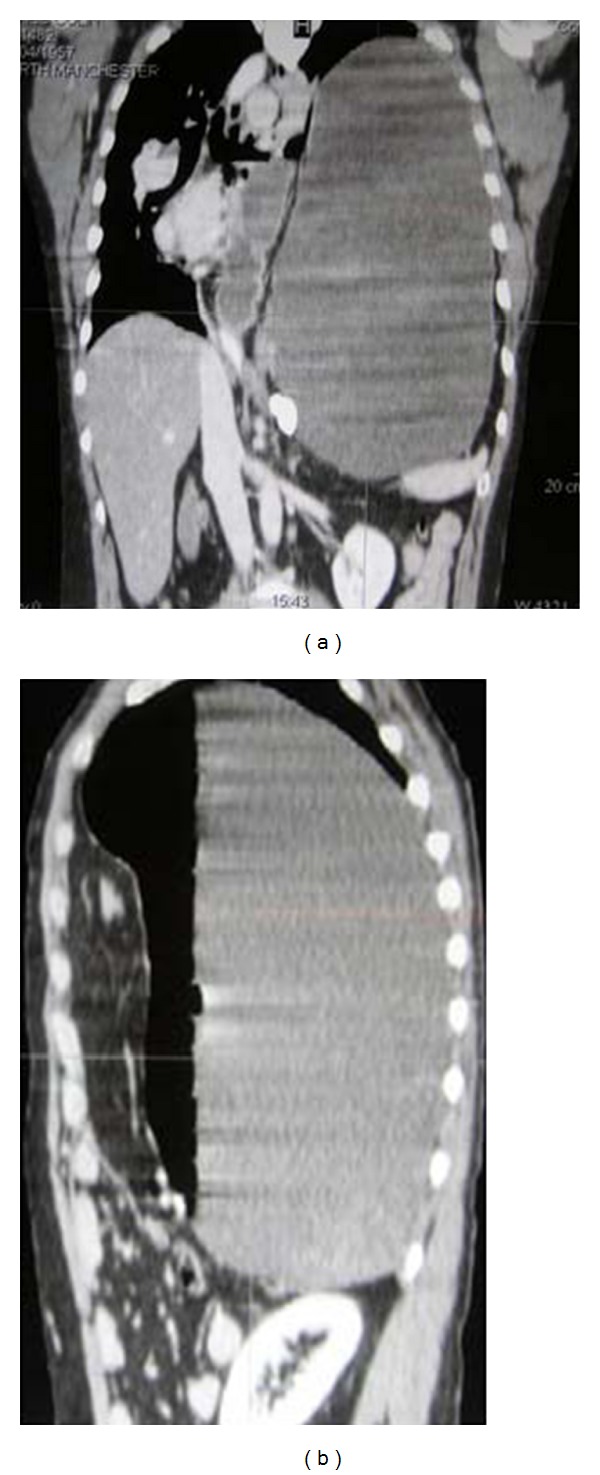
Coronal and sagittal CT reconstruction on the same patient as Figures 13, 14, and 15 showing diaphragmatic rupture and herniation of the stomach into the left hemithorax.
